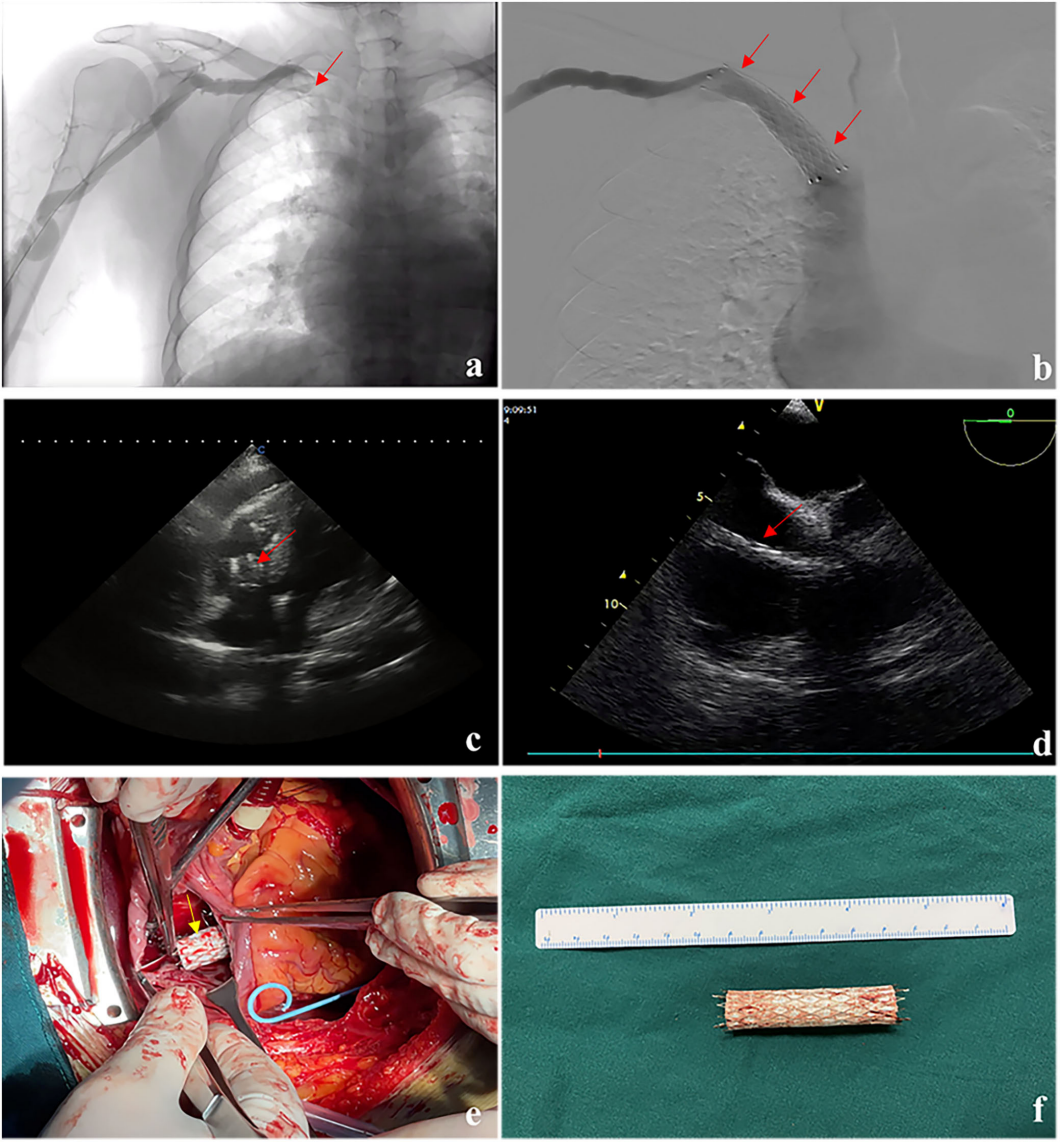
Imaging data and intraoperative data of the patients: (a) stenosis (red arrow) of the confluence of the right subclavian vein and the innominate vein in a digital subtraction angiography examination, (b) stent (red arrow) placement in central vein stenosis, (c) transthoracic echocardiography showing pericardial effusion and a stent (red arrow) in the right ventricle, (d) transesophageal echocardiography indicating a segment of stent migration (red arrow) in the right ventricle, (e) the tail of the stent (yellow arrow) was found to have been inserted into the atrial wall during the operation, and (f) a retrieved covered stent.
